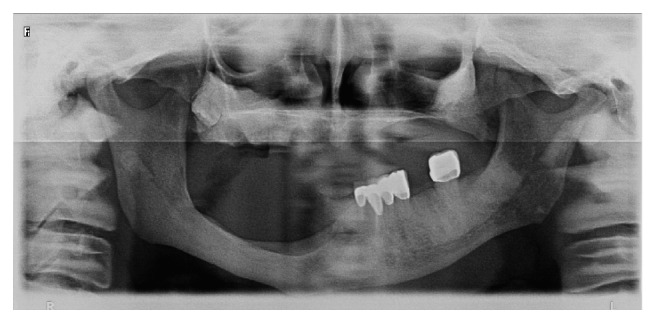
Panoramic radiography of patient at 1-year follow-up visit in 2015.
